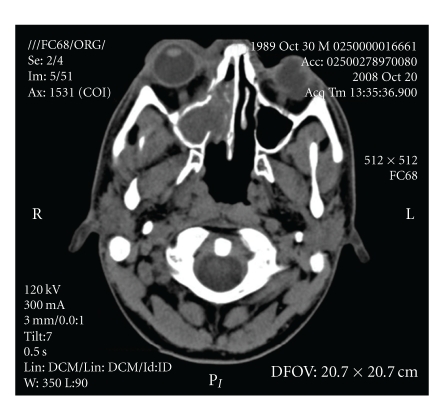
CT scan of the perinasal sinuses: infiltrative lesion of the perinasal sinuses that exerts compression on the right optic nerve.
